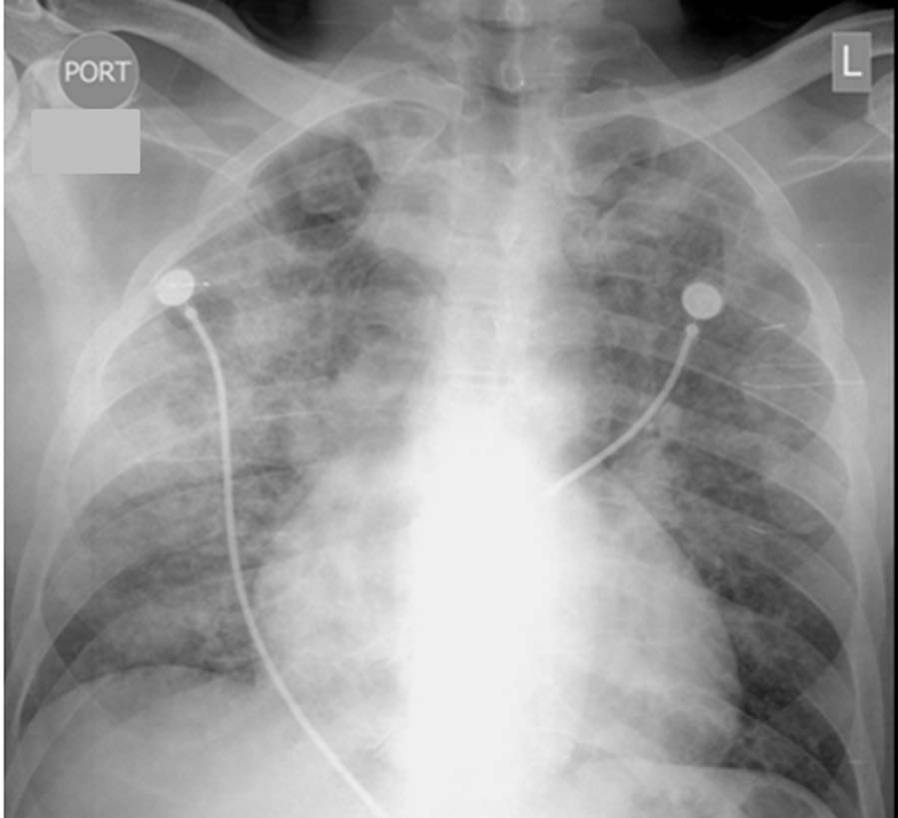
Chest radiograph showing bilateral interstitial-alveolar pattern.
